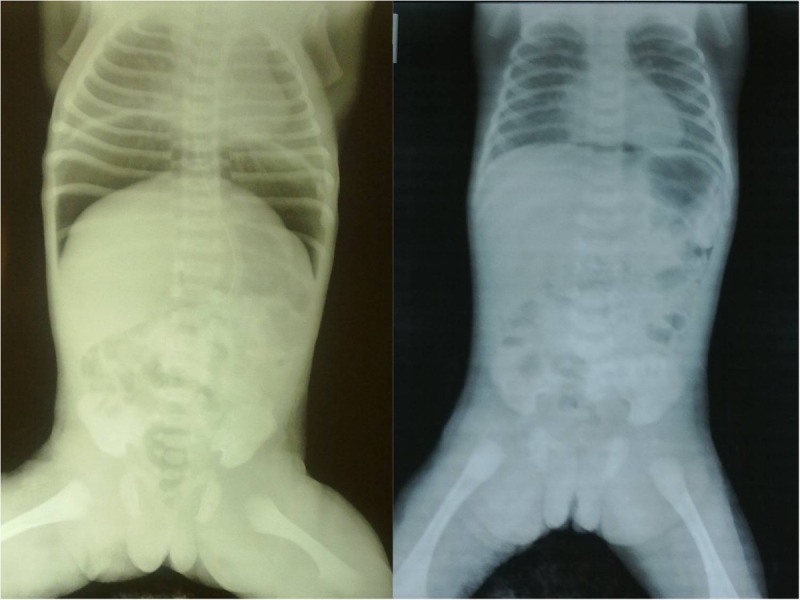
Figure 2: (a) Radiographs revealing the pneumoperitoneum (left). (b) Radiographs revealing resolution of spontaneous pneumoperitoneum after conservative management (right).
